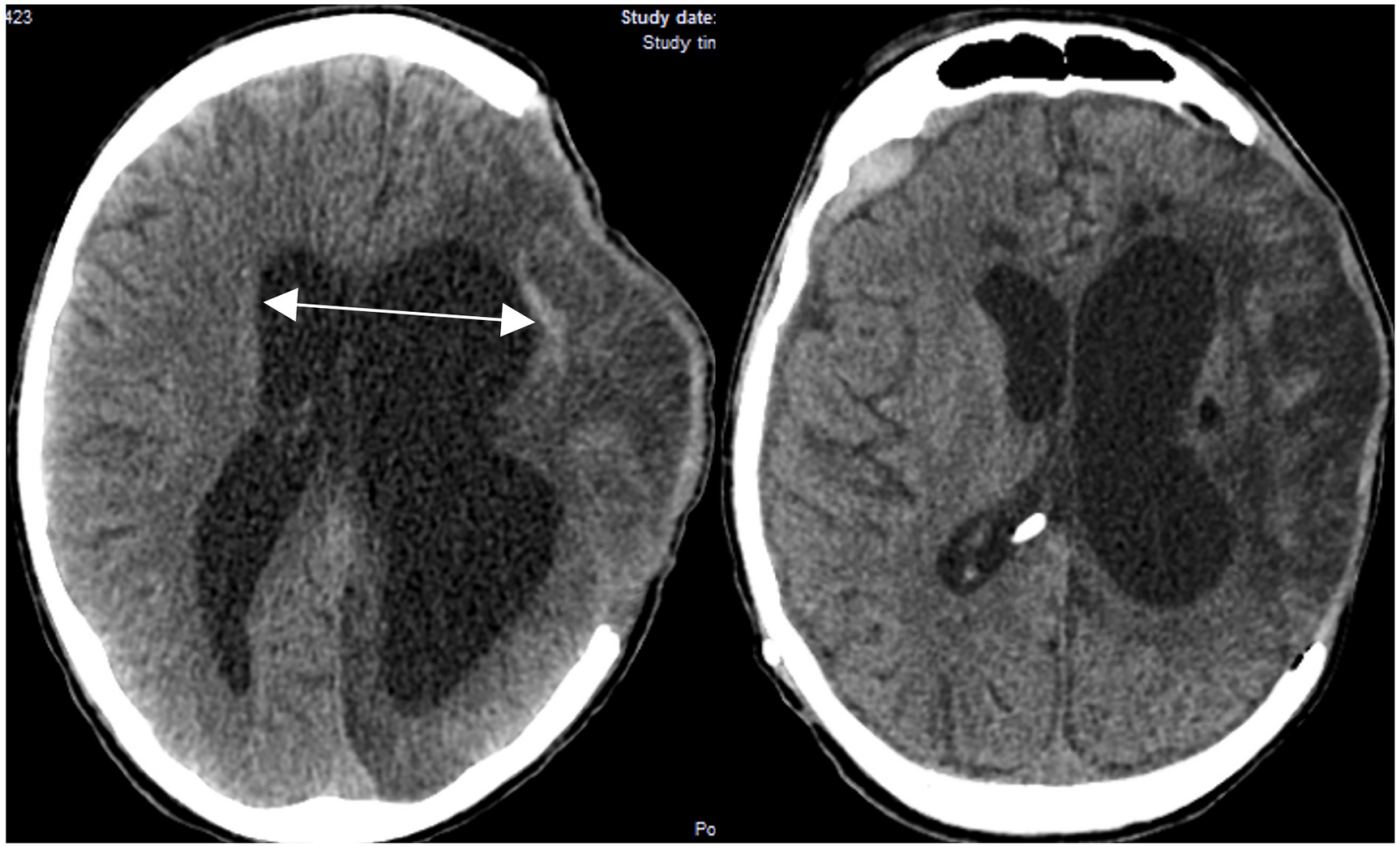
CT brain showing posttraumatic hydrocephalus after decompressive craniectomy.
